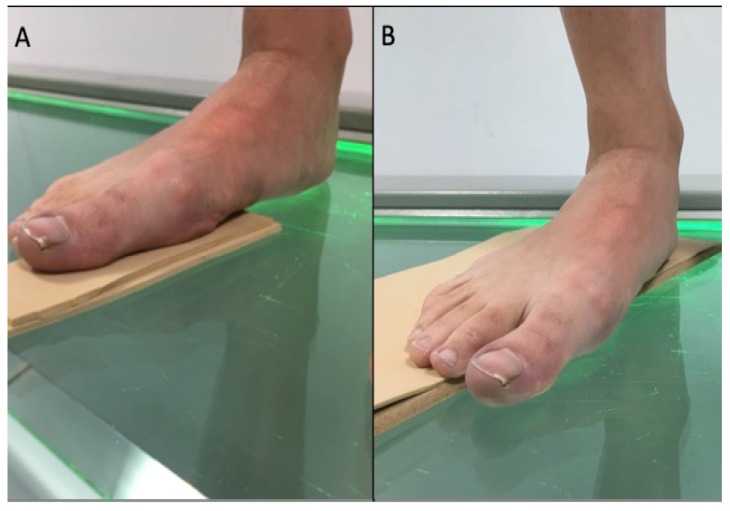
Foot positioning for AP radiographs (A): First ray dorsiflexion; (B): First ray plantarflexion.
